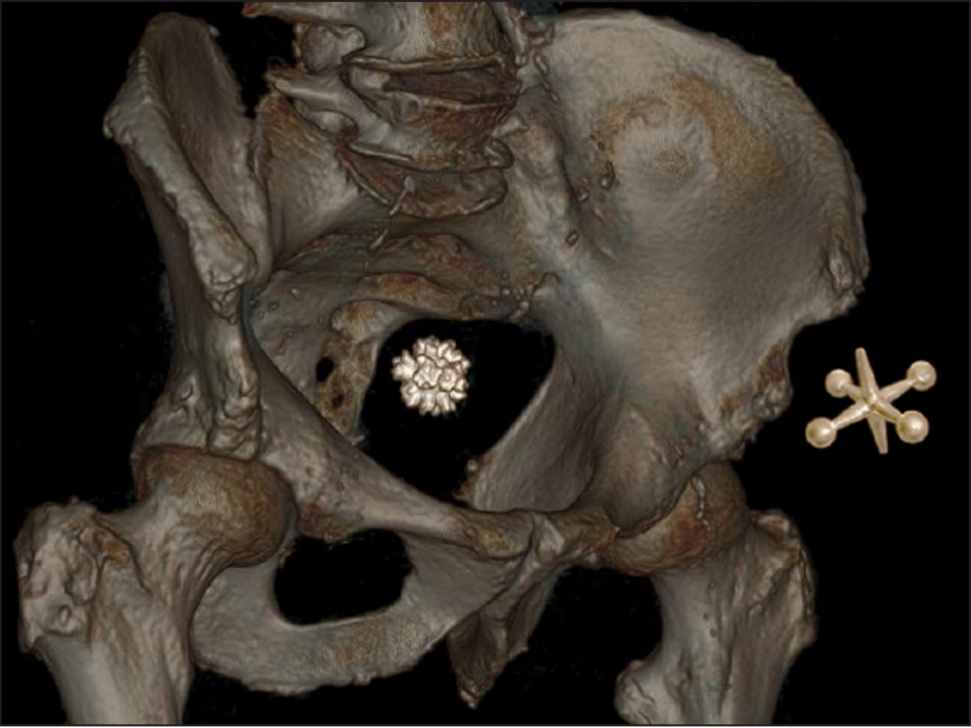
3D reformat of the non-contrast CT scan, with added real-size 3D model of a Jack toy.
